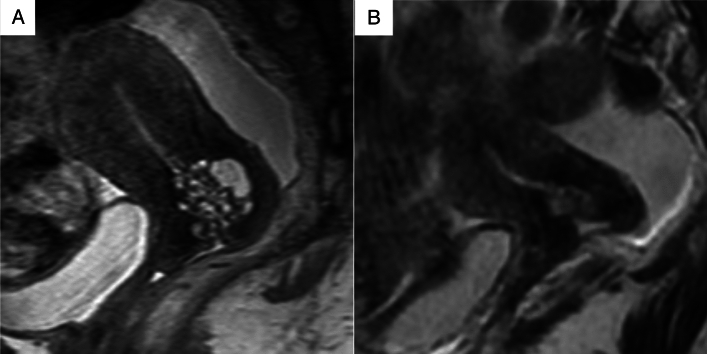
A Sagittal T2-weighted (T2WI) image of a 55-year-old woman (premenopausal) with atypical lobular endocervical glandular hyperplasia showing a cystic aggregate lesion in the upper part of the cervix, with some small cysts and solid components in the central part of the lesion, surrounded by relatively large cysts. This lesion was classified as the “typical cosmos pattern.” B Follow-up of this patient at the age of 63 years (postmenopausal) shows remarkable shrinking of the lesion and the typical cosmos pattern changed to a microcystic pattern
